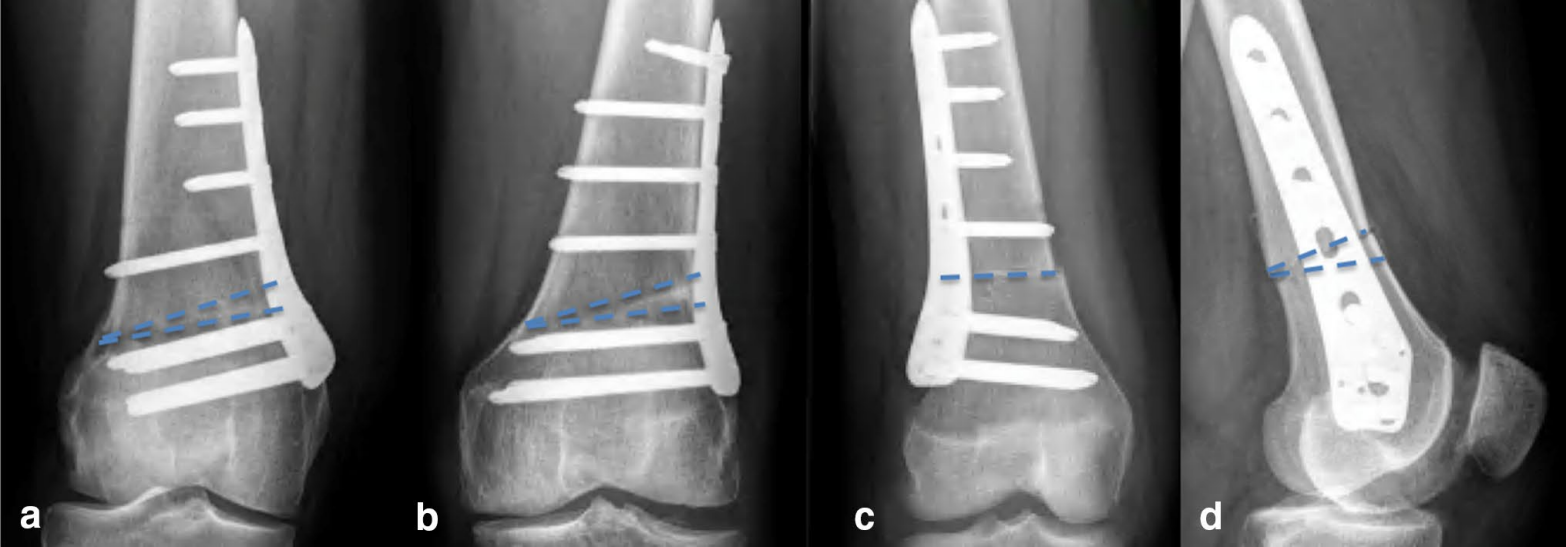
Posteoperative anteroposterior/lateral radiographs of distal femoral osteotomies (DFOs) with projected osteotomy cuts (striped lines). a Right knee after medial closing wedge DFO, b Left knee after lateral closing wedge DFO, c Right knee after de-rotation DFO, d Left knee after anterior closing wedge DFO
